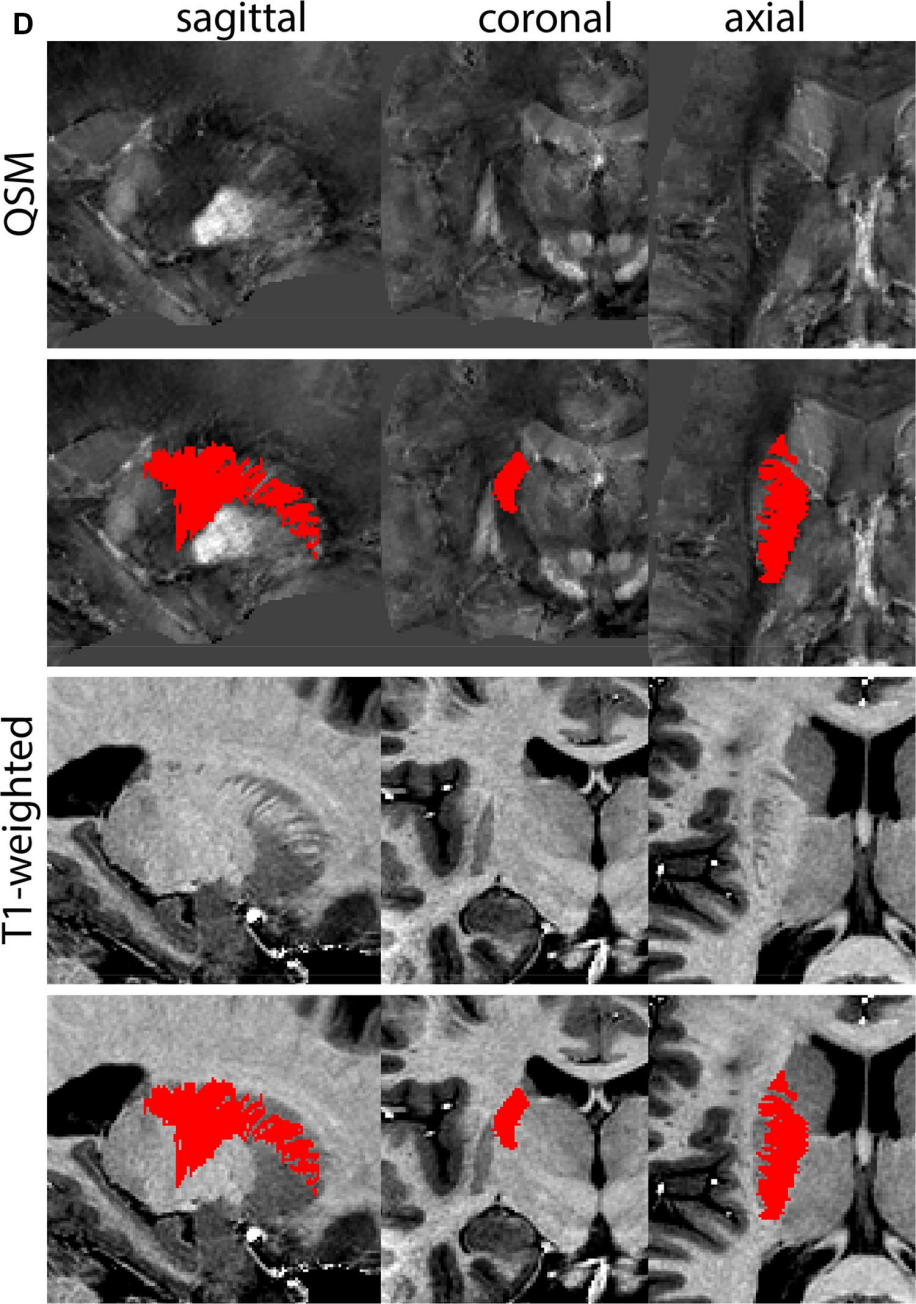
a The internal capsule (ic) is a white matter structure that separates the caudate nucleus from the dorsal striatum, carrying from and to cerebral cortex. Note the limited contrast in the T1-weighted scans between ic and the Globus Pallidus, and the additional contrast provided by the QSM contrast. b The internal capsule (ic) is a white matter structure that separates the caudate nucleus from the dorsal striatum. Note the clear appearance of striatal islands that lead to a jagged appearance of the ic. c The internal capsule (ic) is a white matter structure that separates the caudate nucleus from the dorsal striatum, carrying from and to cerebral cortex. d The internal capsule (ic) is a white matter structure that separates the caudate nucleus from the dorsal striatum, carrying from and to cerebral cortex
